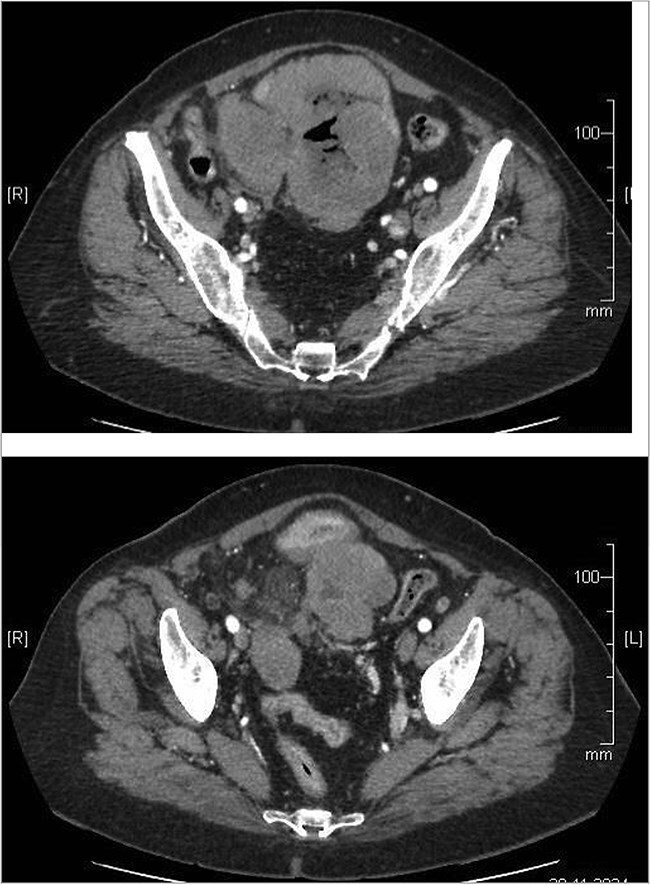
Abdominal CT showing small bowel tumor and similar to both ovaries.
